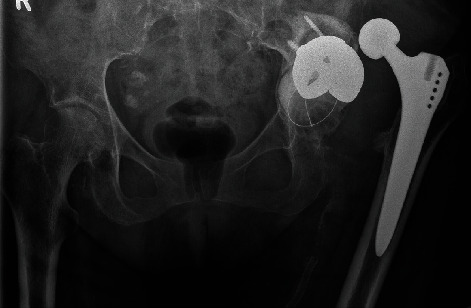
Follow-up X-ray demonstrating left hip dislocation at 4 weeks postop.
